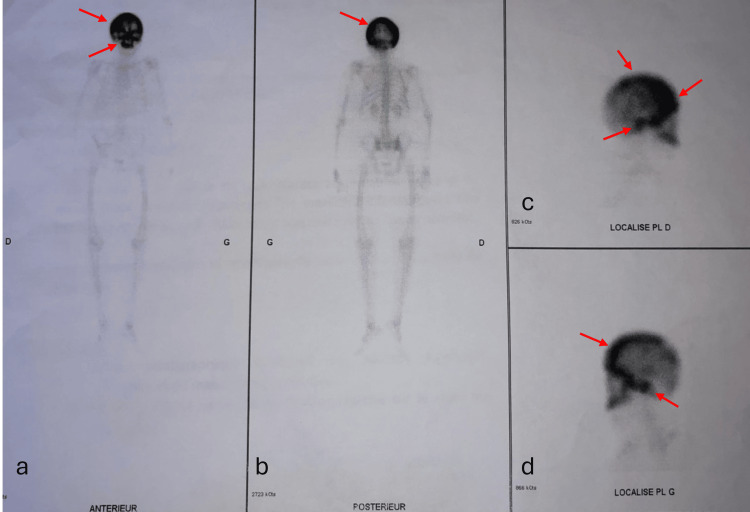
Total bone (a,b) and skull (c,d) scintigraphy Increased radionuclide uptake exclusively in the skull and facial massif (red arrows).
